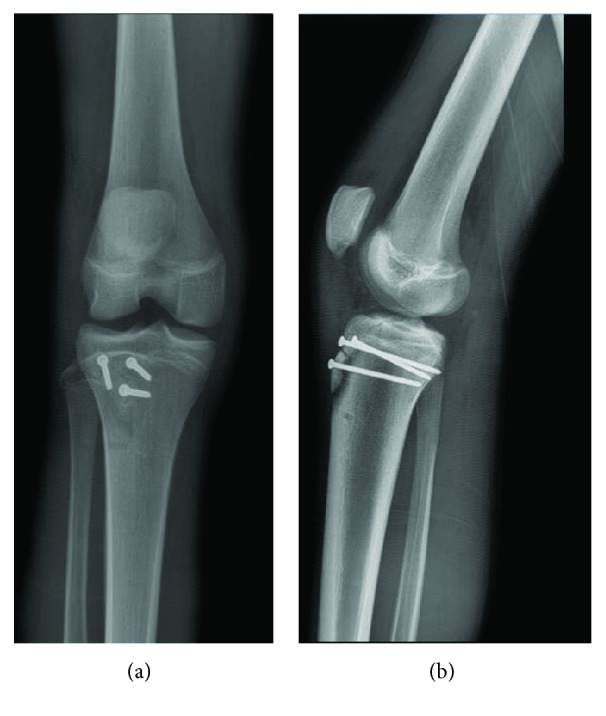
Anterior-posterior (a) and lateral (b) X-rays 2.5 weeks following operative management demonstrates well-positioned screws with callus formation beginning at the site of injury.
